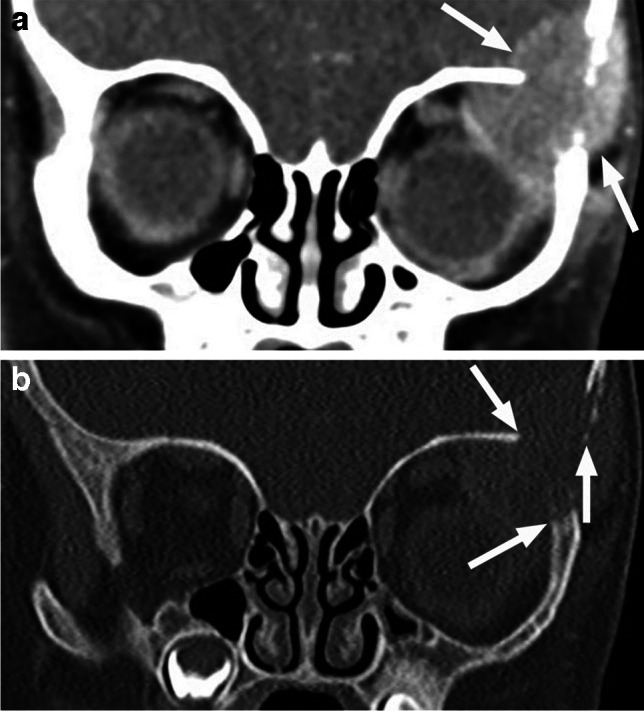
Coronal postcontrast CT images in soft tissue (a) and bone (b) window and algorithm show a destructive lesion of the left superolateral orbital wall with soft tissue components exhibiting slightly more intense peripheral enhancement (arrows in a) and relatively well-defined bony margins (arrows in b) in this patient with Langerhans cell Histiocytosis (LCH)
